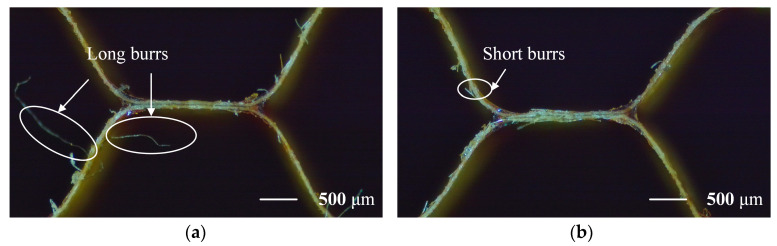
(a) Burr length without UV, feed rate, and spindle speed at 3000 mm/min and 3000 r/min, respectively. (b) Burr length with UV, ultrasonic amplitude at 20 μm, spindle speed at 3000 r/min, and feed rate at 3000 mm/min.
